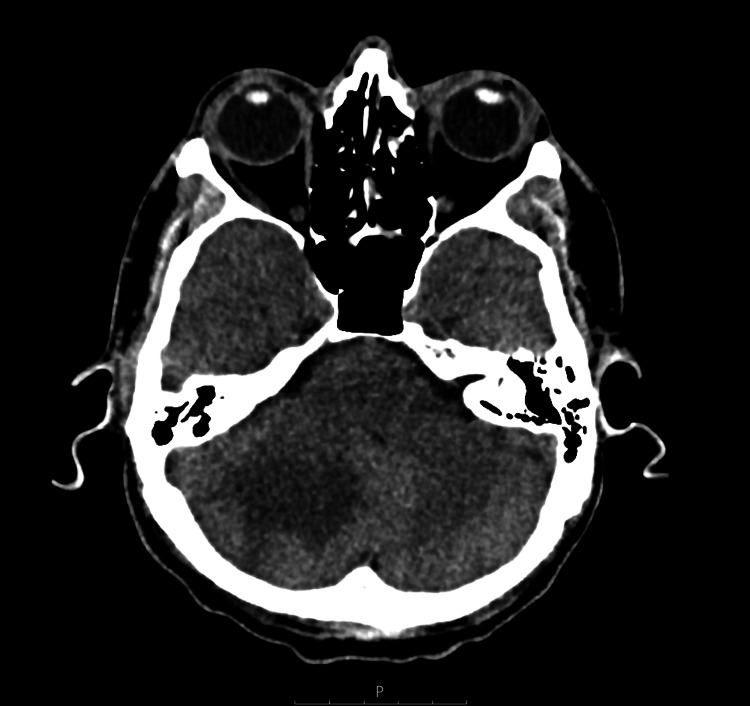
Non-contrast CT head reconstruction showing a focal area of low attenuation within the right cerebellar hemisphere
